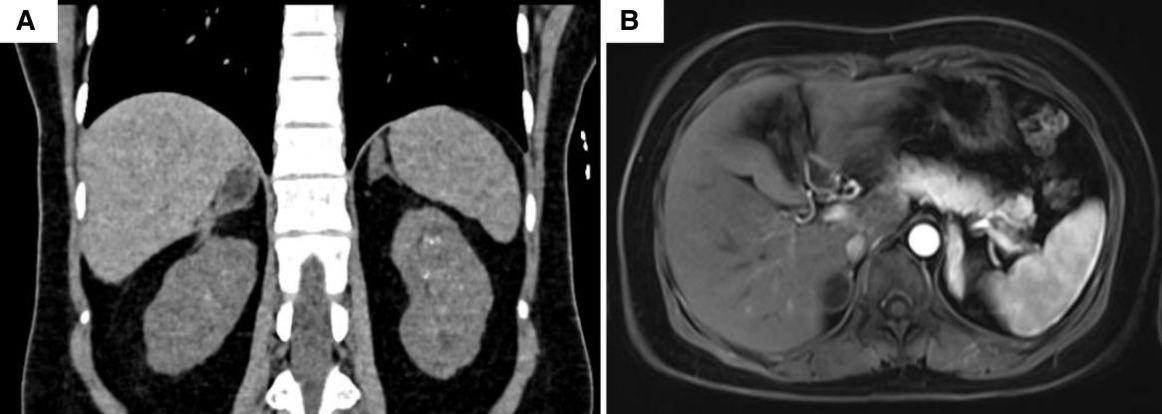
Coronal unenhanced computed tomography of the upper abdomen. The scan shows fat (HU: −27) containing myelolipoma in the right adrenal gland with hyperplasia of the limbs, hyperplasia of the left adrenal gland, medullary nephrocalcinosis in both the kidneys with a few small medullary cysts in the left kidney (A), and axial contrast enhanced T1-weighted fluid-attenuated inversion recovery sequence magnetic resonance imaging showing a fat-containing lesion in the right adrenal gland, suggestive of myelolipoma (2.3 × 1.7 × 2.6 cm), and homogenously enhancing hyperplastic left adrenal gland.
